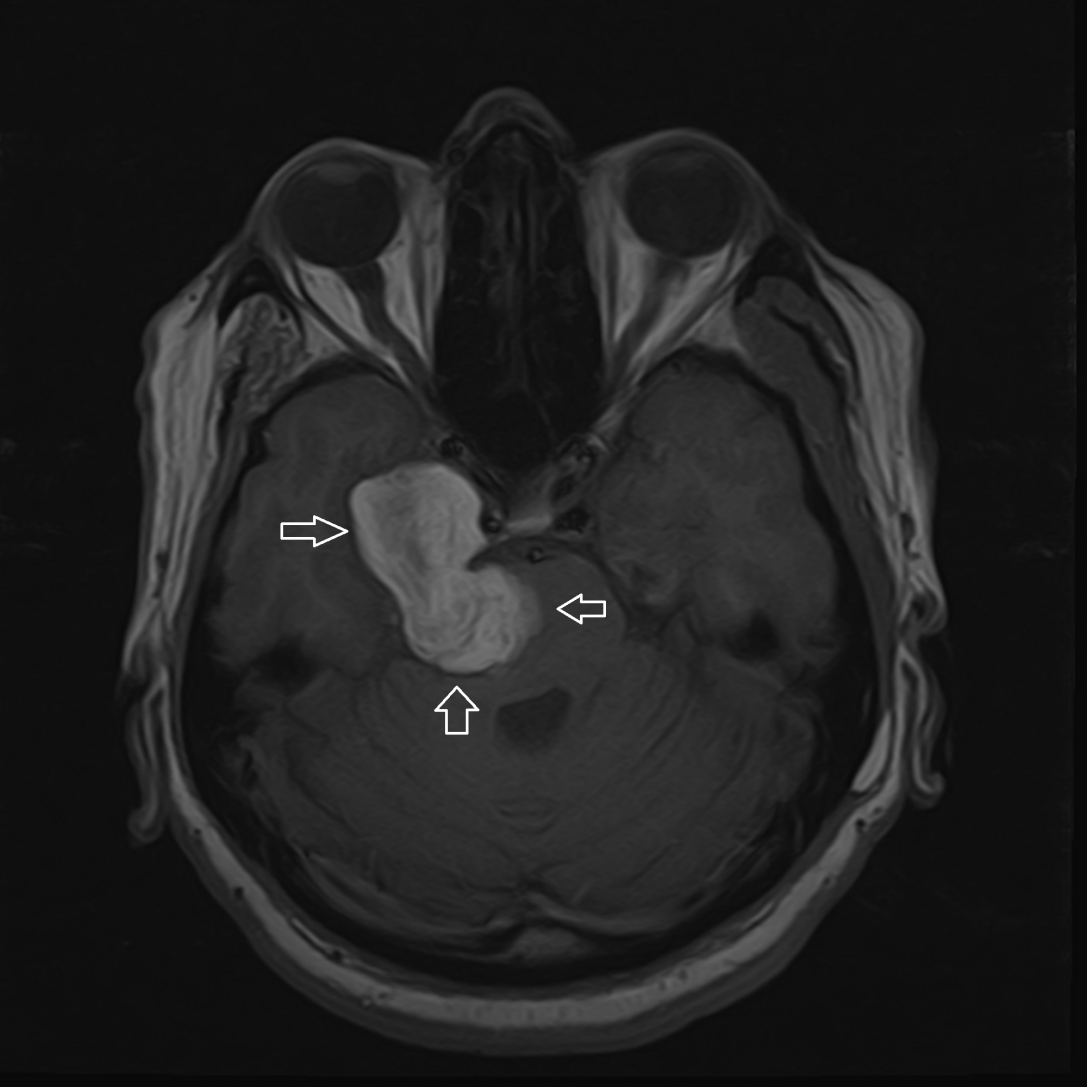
MRI T1 axial brain non-contrast T1 hyperintense lobulated extra-axial mass based on middle and posterior cranial fossae
